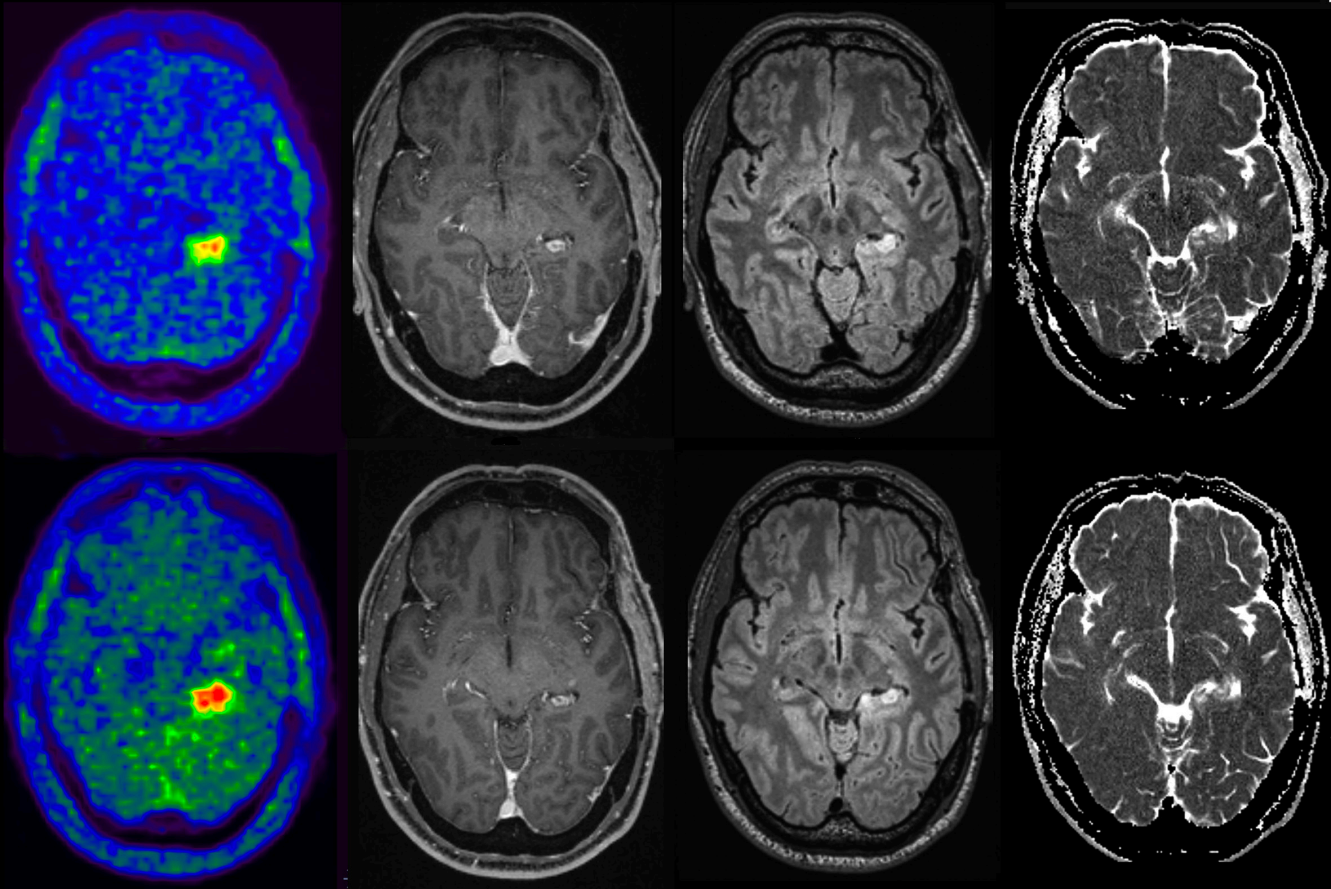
Axial [^18^F]FET PET/MR images before (upper row) and after (lower row) treatment with regorafenib in one of the study patients (#5). first column: [^18^F]FET PET; second column: post-contrast *T*_1_ weighted; third column: FLAIR; fourth Column: ADC maps. The increase in [^18^F]FET uptake after treatment with regorafenib is evident in lower row first column compared with upper row even though no significant increases in the contrast-enhanced and FLAIR altered areas are visible. The analysis revealed an increase in the pathological ADC-volume after regorafenib, even though it was barely detectable qualitatively on the native images. ADC, apparent diffusion coefficient; FET, *O-*(2-18F-fluoroethyl)-L-tyrosine; FLAIR, fluid attenuated inversion recovery; PET, positron emission tomography.
